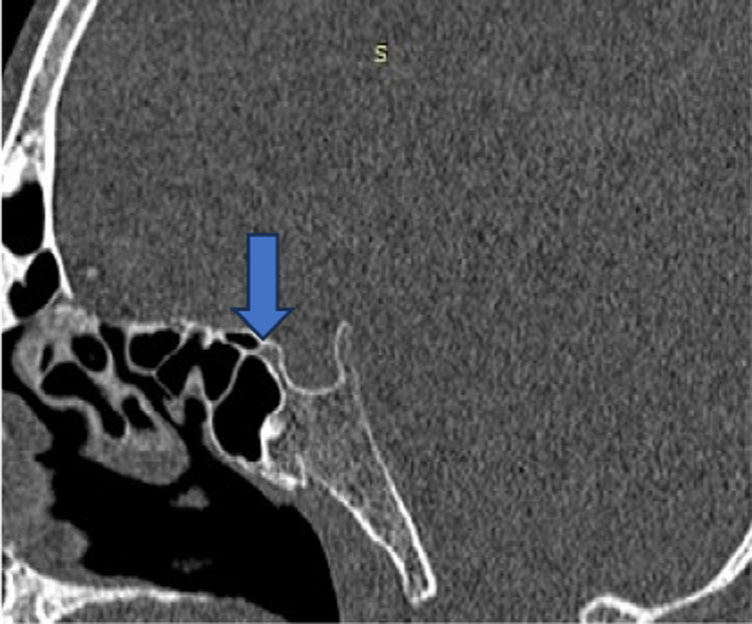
CT Sagittal image shows presellar pneumatization of sphenoid sinus.
